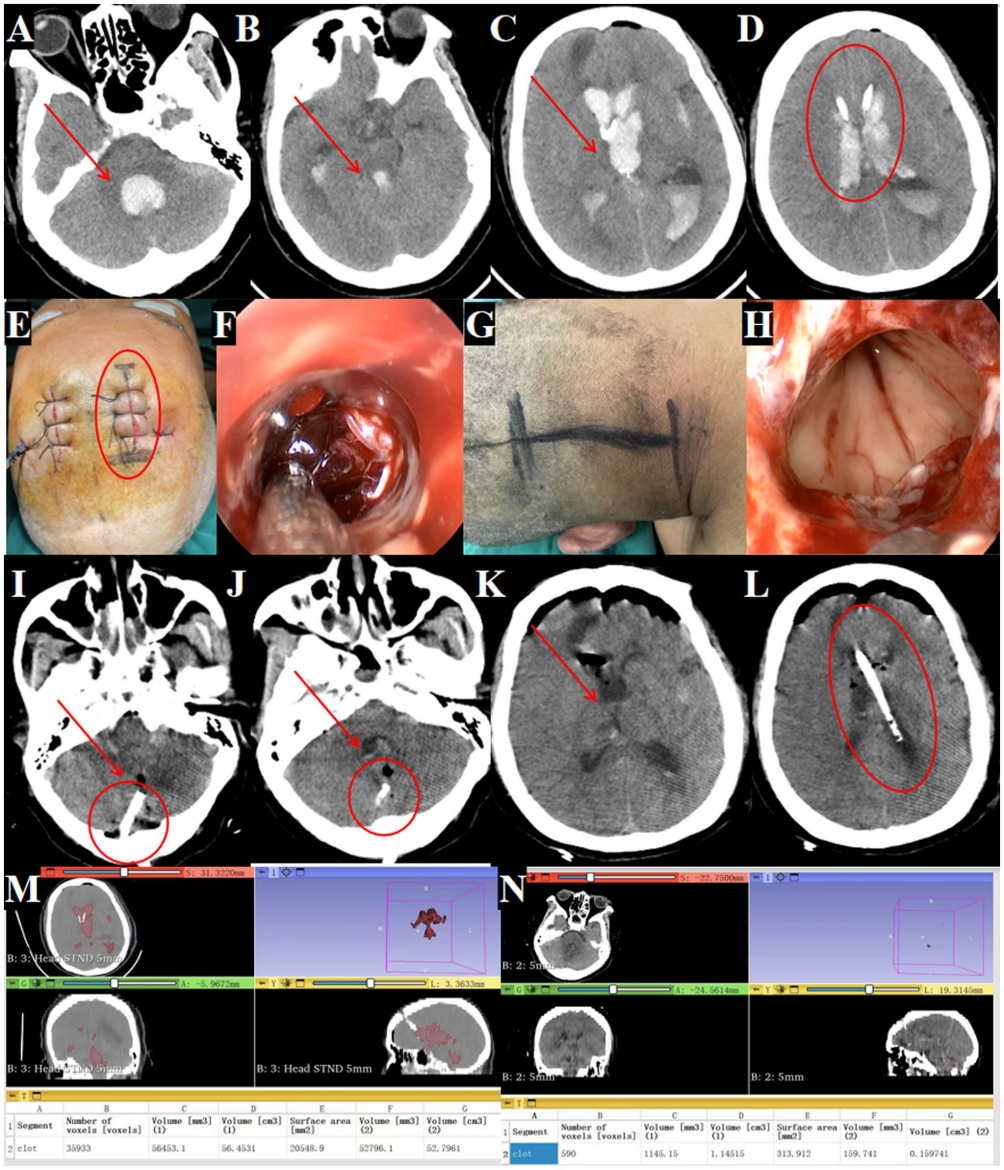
Management of fourth ventricular hematoma requiring dual surgical approaches. (A–D) Post-transfer cranial CT after EVD at a local hospital (→ arrows in A–C show residual hemorrhage in the fourth, third and lateral ventricles; ◯ circle in D marks the external drainage site). (E,F) Supratentorial approach for hematoma removal (◯ circle indicates entry point). (F) Evacuation of hematoma in the lateral and third ventricles. (G) Infratentorial approach for fourth ventricular hematoma removal. (H) Evacuation of hematoma in the fourth ventricle. (I–L) Postoperative CT confirming complete hematoma clearance (→ arrows denote evacuated ventricles; ◯ circles show drainage tubes). (M) Preoperative hematoma volume: 56.4 mL. (N) Postoperative hematoma volume: 1.1 mL.
